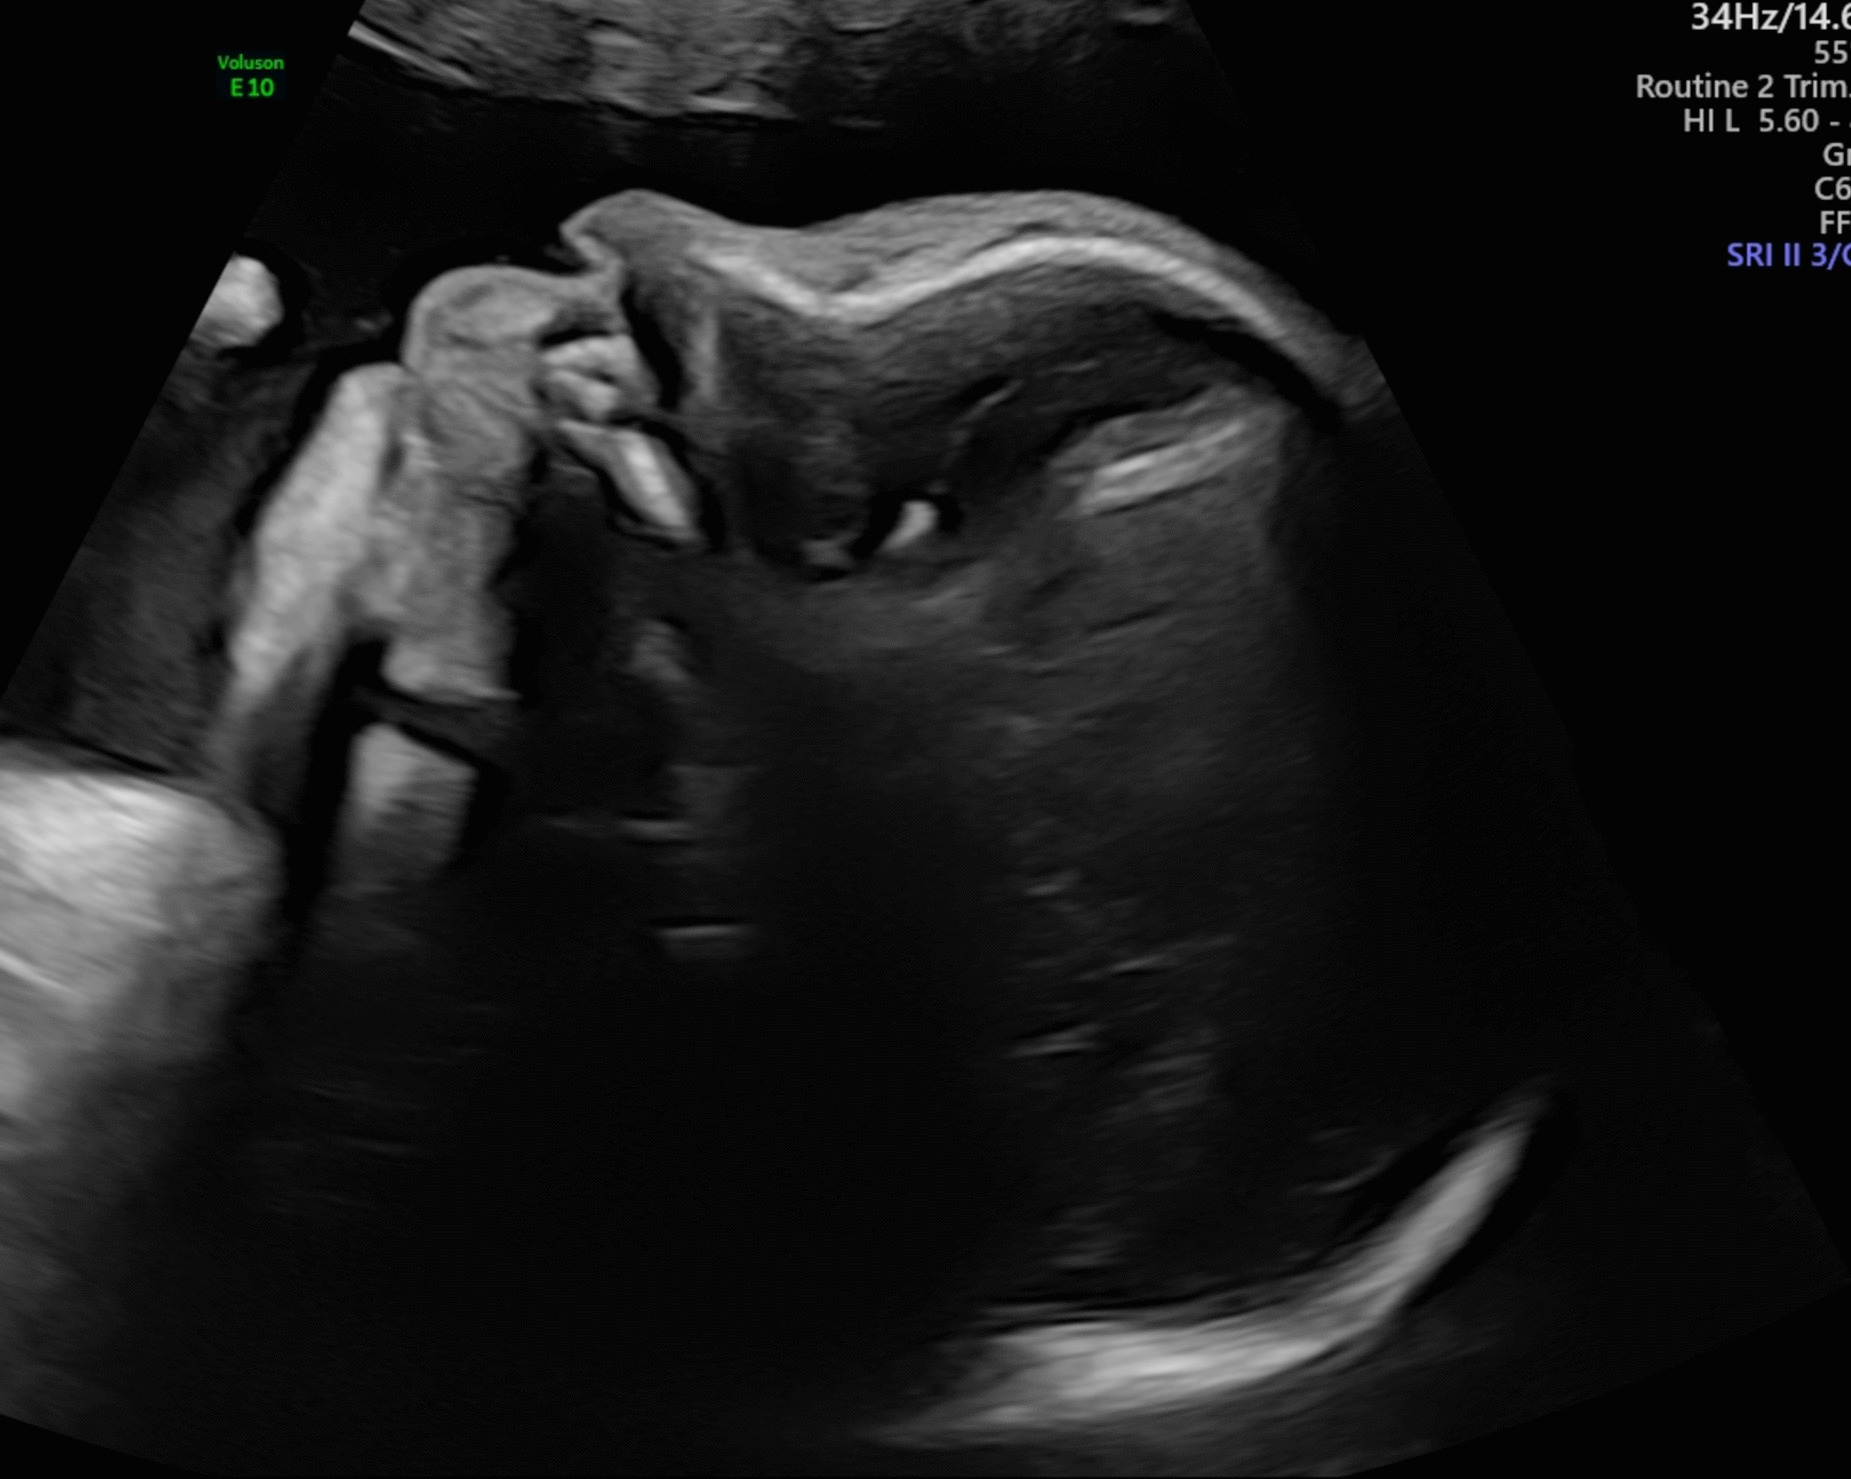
Ultrasound at 31 weeks showing micrognathia
